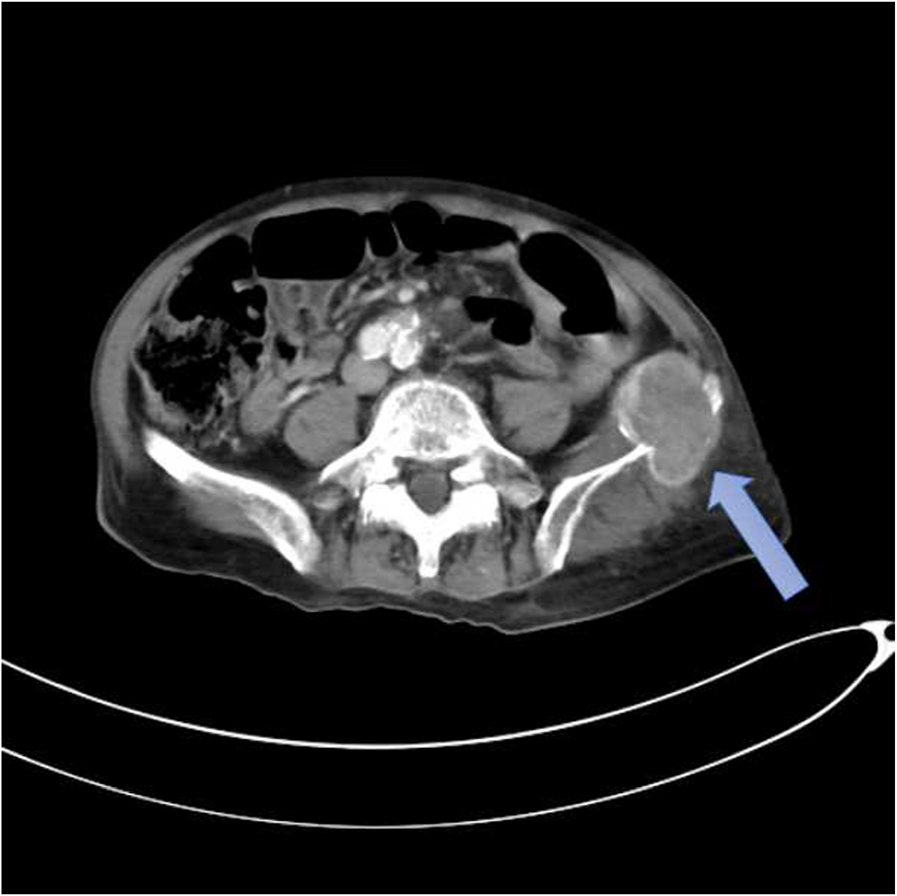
Axial view of contrasted computed tomography scan of the pelvis shows a lytic lesion at the left ilium (*blue arrow*)
